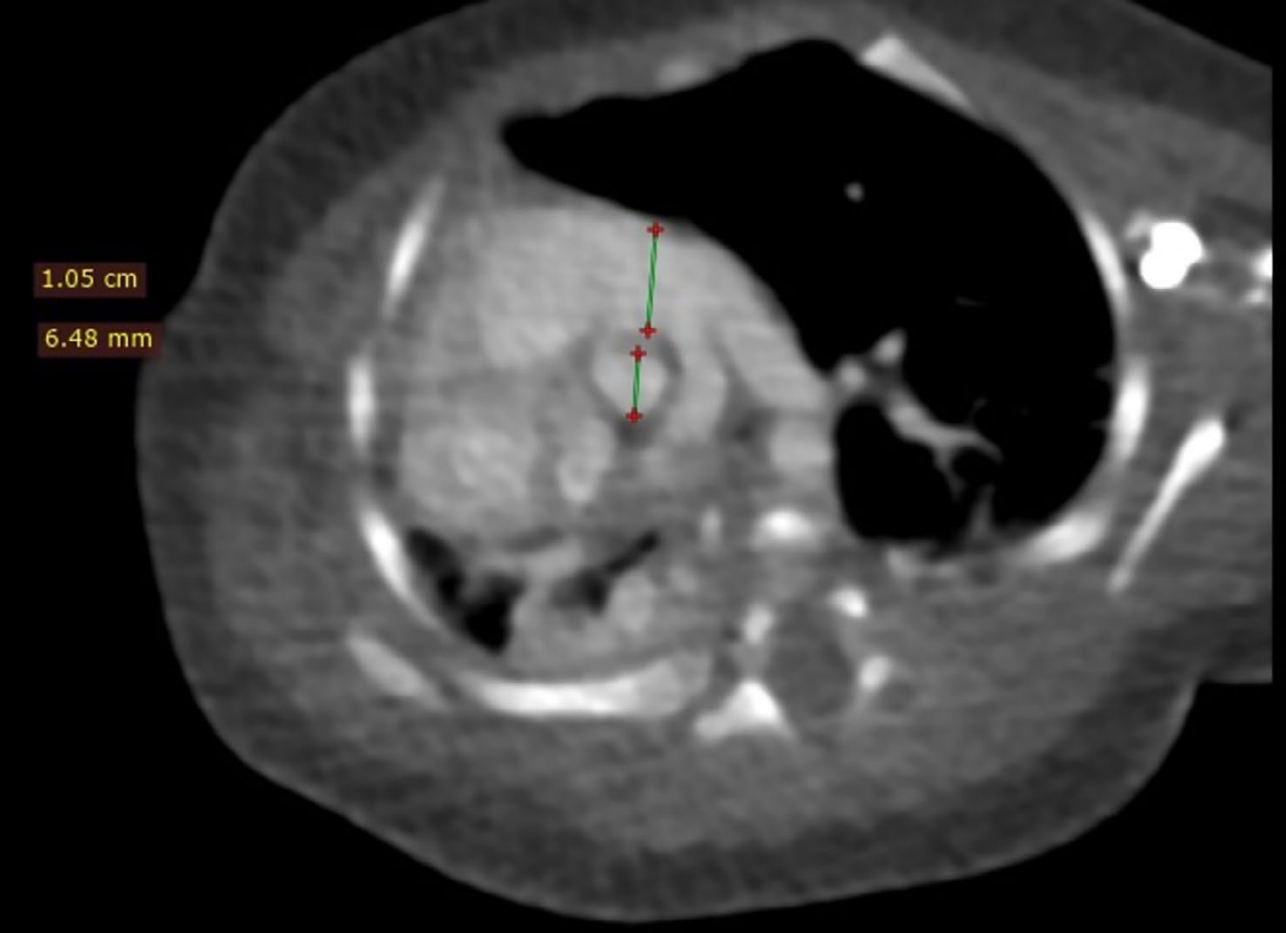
A post IV contrast CT of the chest, an axial slice at the level of the great vessels, soft tissue window which is demonstrating a visibly enlarged main pulmonary trunk with a pulmonary trunk to aortic ratio of more than 1.6. The right pulmonary artery is also slightly smaller than the left pulmonary artery. With the exception of right‐sided rib crowding, there were no overlying bony structural defects noted.
